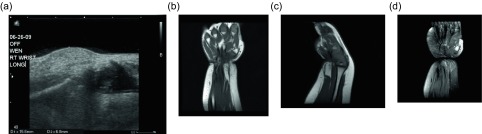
Lipoblastoma: 1-year-old girl with a right wrist mass. Directed ultrasound (a) of the right wrist portrays a well-circumscribed, predominately hyperechoic lesion in the superficial soft tissues. Coronal (b) and sagittal (c) T1W pre-contrast images show a predominately fat-signal lesion centered around the distal flexor carpi radialis tendon with internal low signal intensity components. T1W FS post-gadolinium (d) images demonstrates enhancement of the internal curvilinear components due to the dense capillary network of the myxoid stroma.
